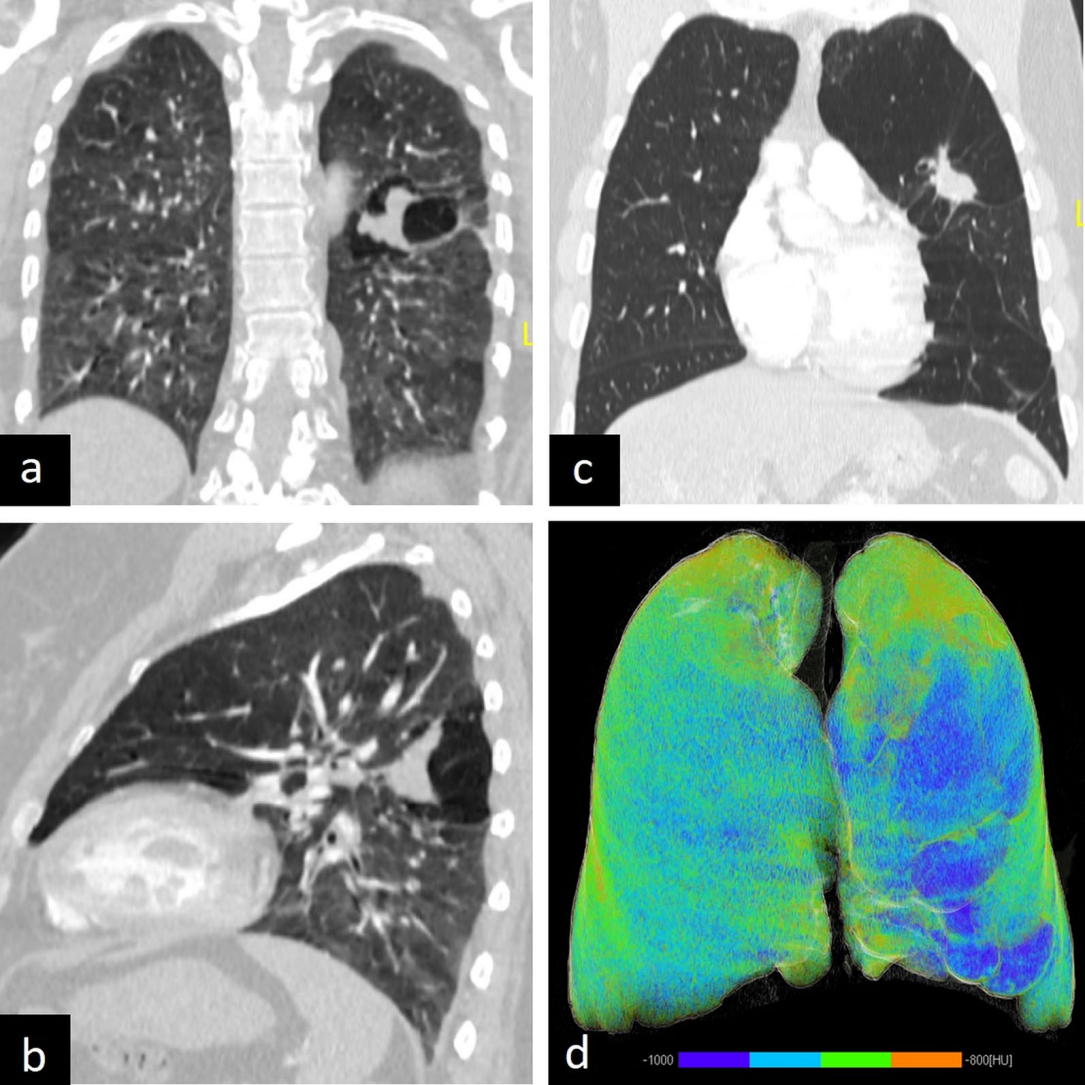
Imaging features of bronchial atresia in two asymptomatic patients. Central mass-like opacity because of the mucus-filled bronchus is seen on coronal (a, c) and sagittal (b) CT images. Coronal (a) and sagittal (b) CT images in the expiratory phase and quantitative 3D reconstruction (d) image clearly show hyperinflation (d, blue area) due to air trapping
